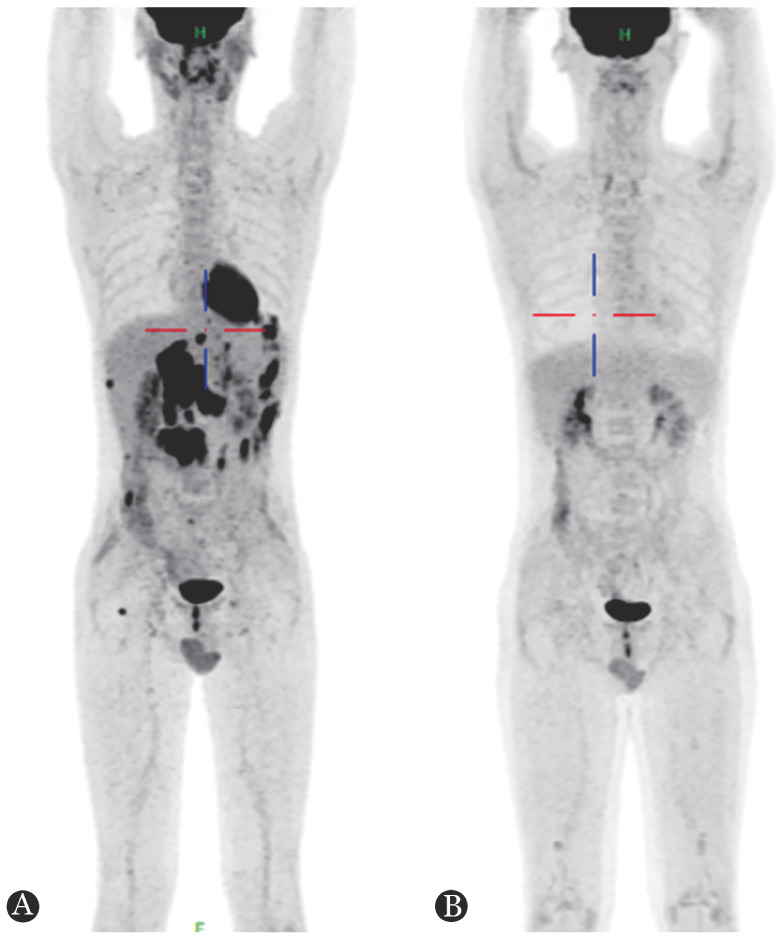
人类免疫缺陷病毒感染相关弥漫大B细胞淋巴瘤患者治疗前后的PET-CT图像 A 确诊淋巴瘤时；B 4个周期R-EPOCH联合PD1方案化疗后
